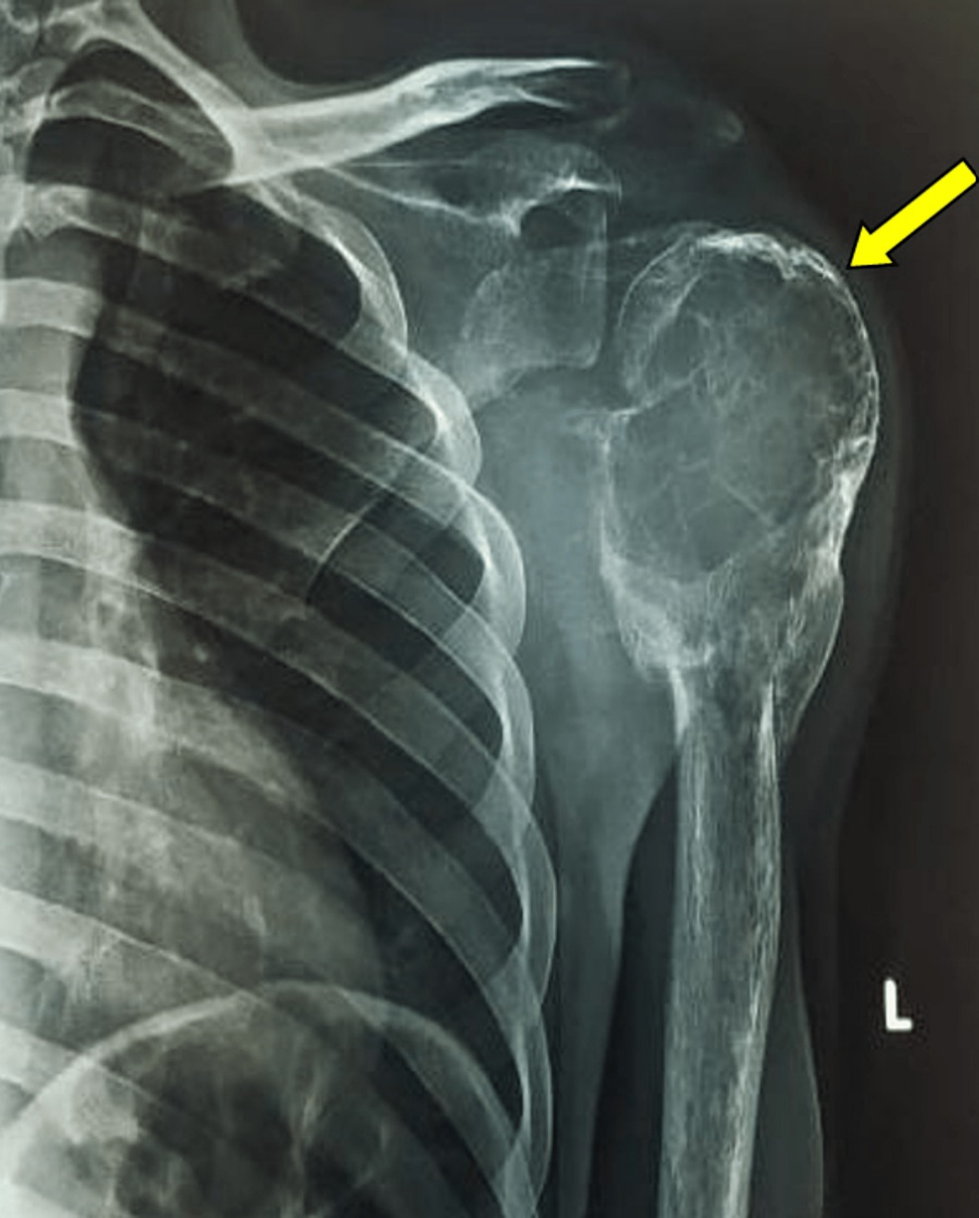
A plain radiograph on the second visit showed an increase in the size of the lesion
